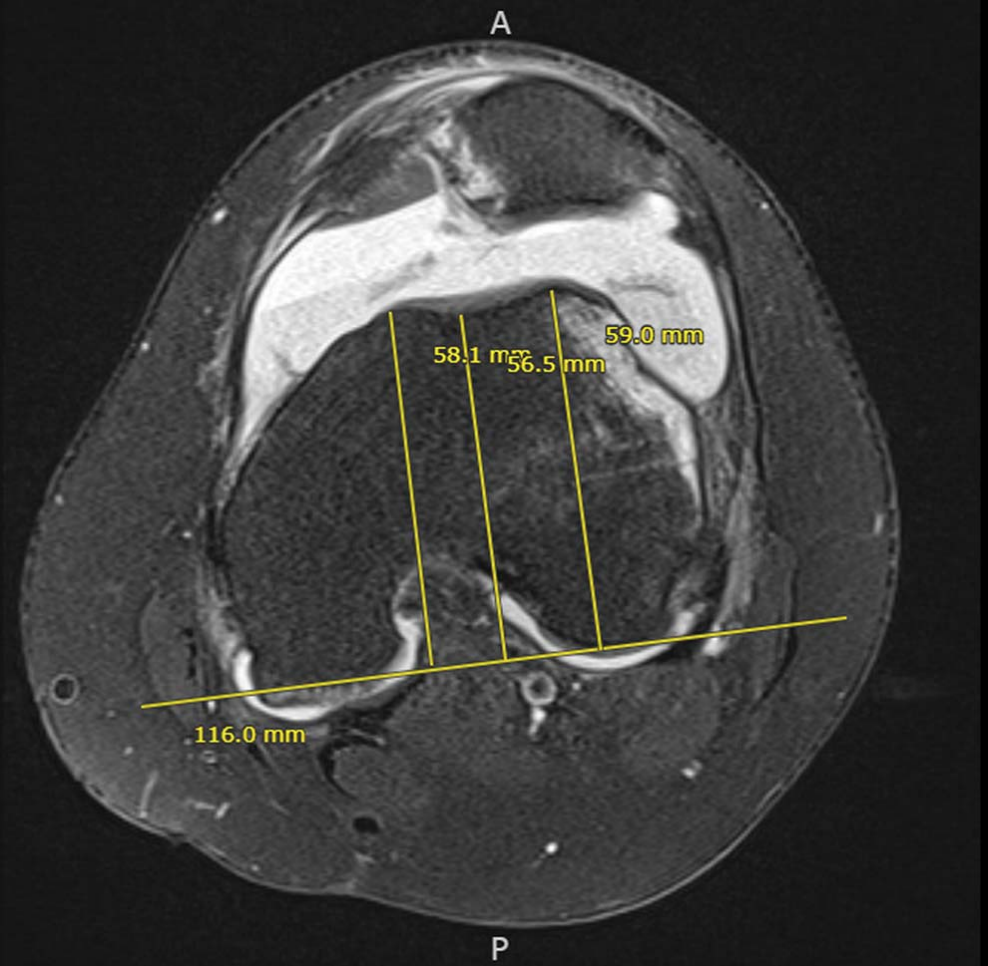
Axial fat-suppressed proton density-weighted magnetic resonance imaging (MRI) shows trochlear dysplasia and the measurements taken for determining the trochlear depth ([59 + 58.1] ÷ 2) – 56.5 = 2.05 mm. An axial MRI 3 cm above the joint line is needed to calculate the trochlear depth. The average distance of the medial and lateral facets from a line tangential to the femoral condyles is subtracted from the distance of the trochlear groove to that same tangential line. A value <3 mm is considered shallow and consistent with trochlear dysplasia.
